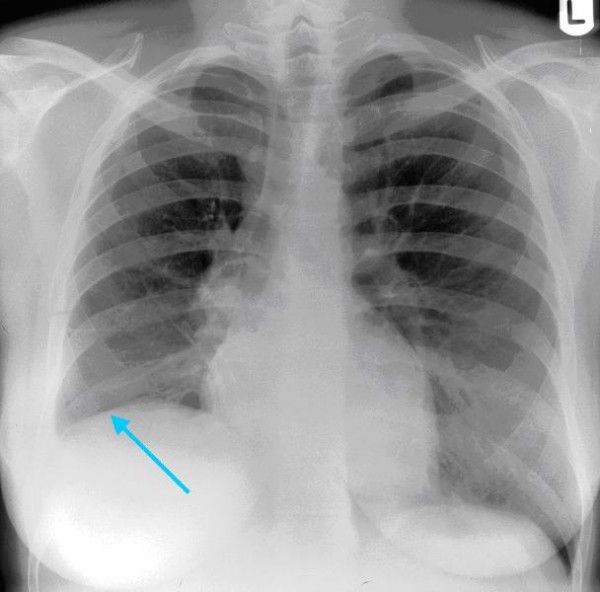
Chest X-ray showing a small right basal pleural reaction and some right basal atelectasis.
